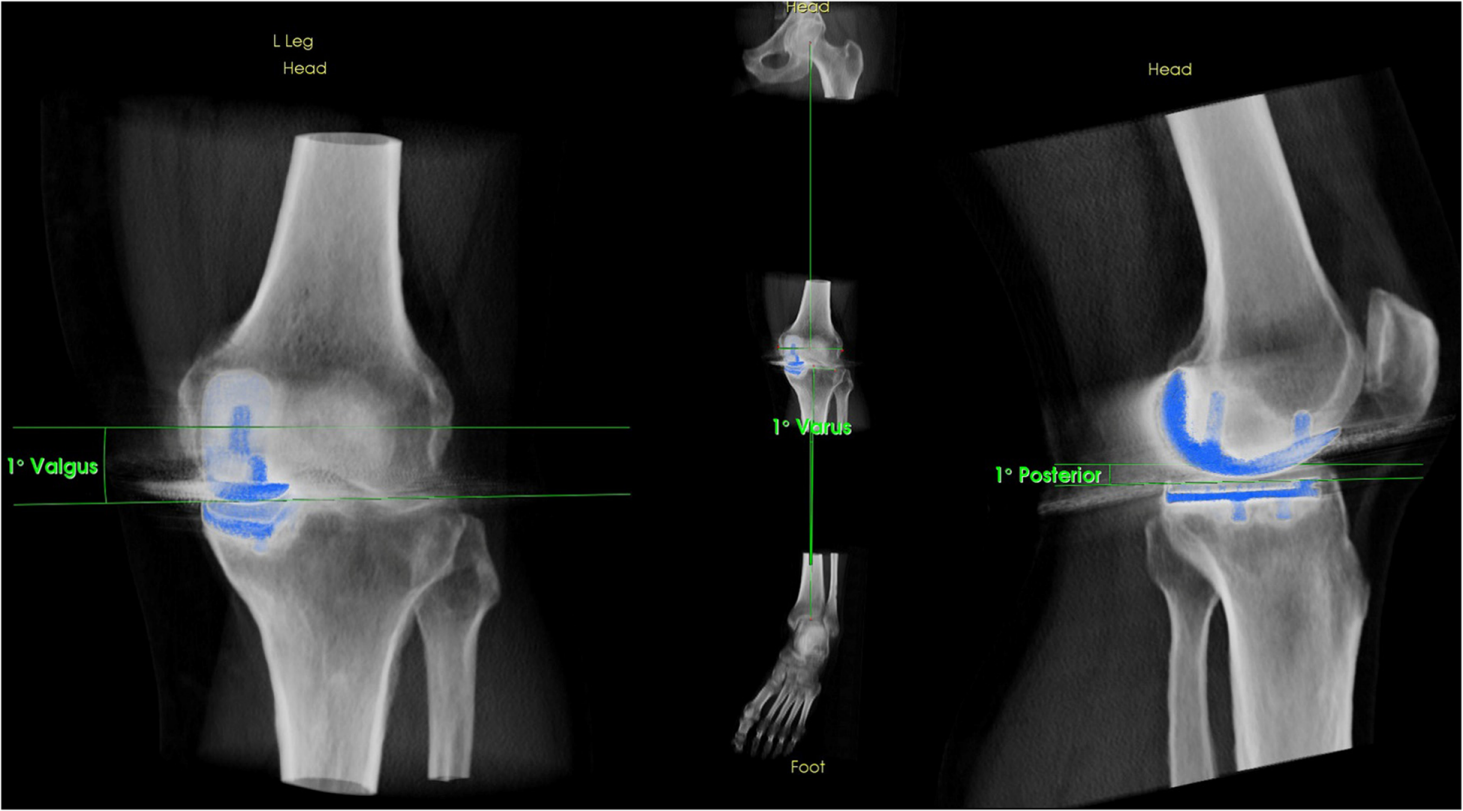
Determination of UKA component position in 3D reconstructed CT images using a customized software.
